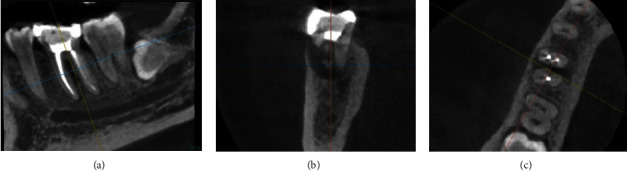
Cone beam computed tomography (CBCT) of Tooth #46 at 6-month follow-up. (a) Sagittal view, (b) coronal view, and (c) axial view.
